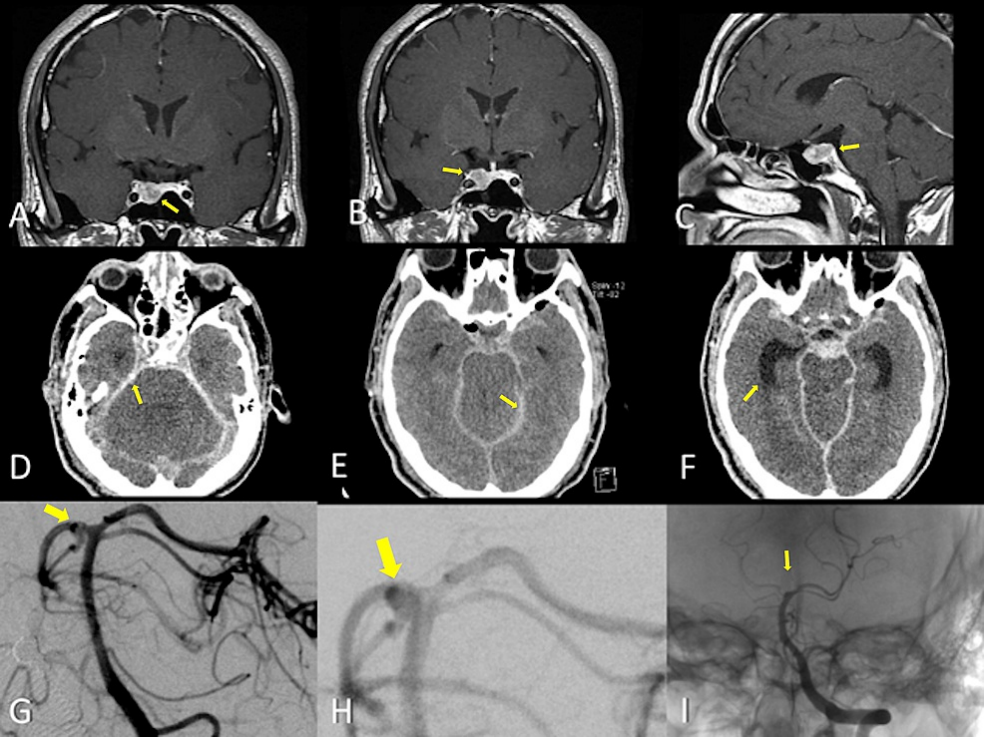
Case 3 representative MRI, CT, and DSA images. (A-C) MRI shows a sellar tumor without retrosellar extension (arrows). (D,E) PO CT scan showing a massive SAH filling all basal cisterns (arrows). (F) Early ventricular enlargement is seen (arrow). (G,H) A small basilar-superior cerebellar artery segment aneurysm is seen (arrow). (I) The patient was coiled for dome protection (arrow). CT, computed tomography; MRI, magnetic resonance imaging; DSA, digital subtraction angiography; PO, postoperative; SAH, subarachnoid hemorrhage.
